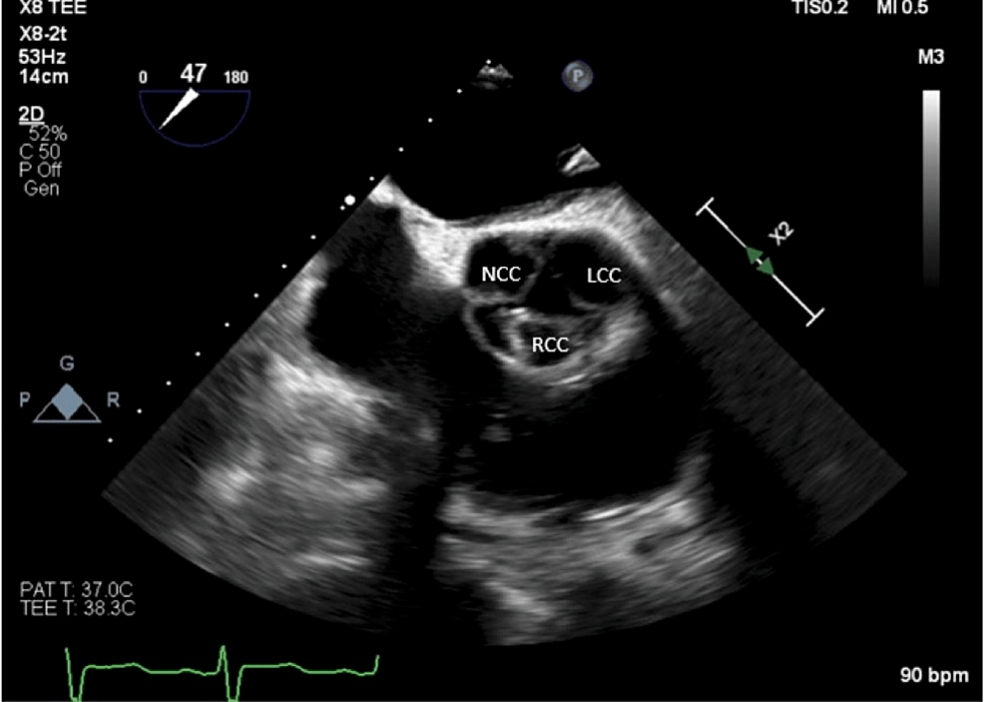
Transesophageal echocardiogram: 2D short axis aortic view at end-diastole The image shows the retraction of the tip of all three leaflets of the aortic valve, particularly the right coronary cusp. NCC - non-coronary cusp; LCC - left coronary cusp; RCC - right coronary cusp
